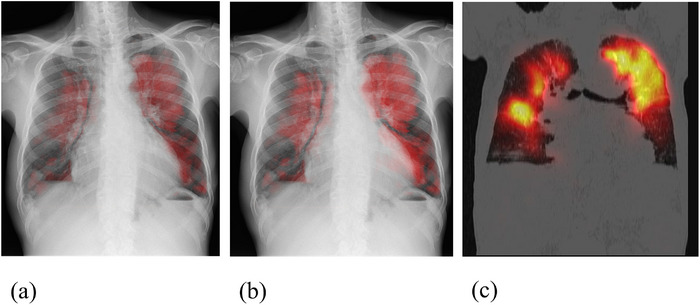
A visual evaluation of the normal blood flow area in a 76‐year‐old male diagnosed with chronic thromboembolic pulmonary hypertension. (a) Maximum intensity projection of low‐signal‐value images. (b) Maximum intensity projection of high‐signal‐value images. (c) Single photon emission computed tomography images used as gold standard.
